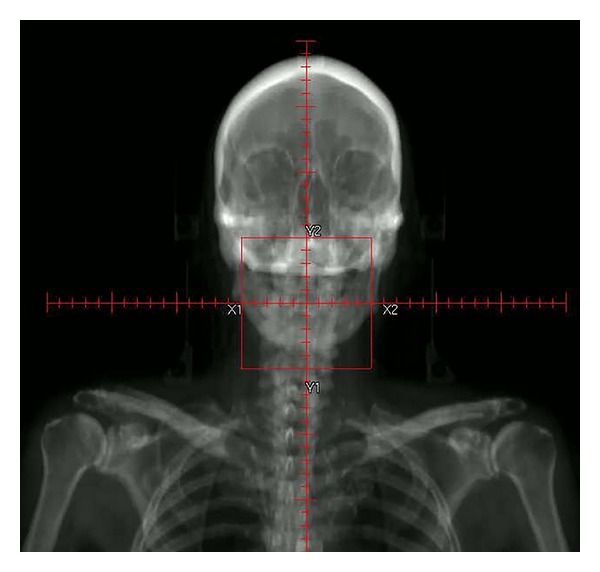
Creation of digital reconstructed radiographs or DRRs from CT.
